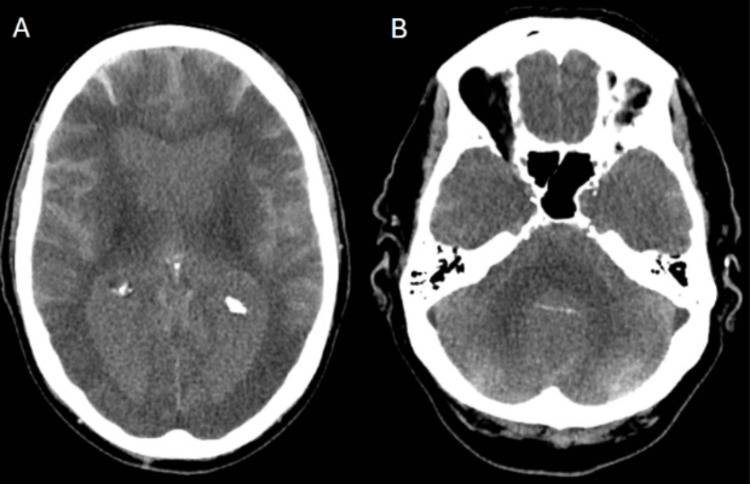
Single-energy CT Axial images (A, B) obtained from single-energy CT of the head, demonstrating hyperdensities in the ventricular and subarachnoid spaces.
